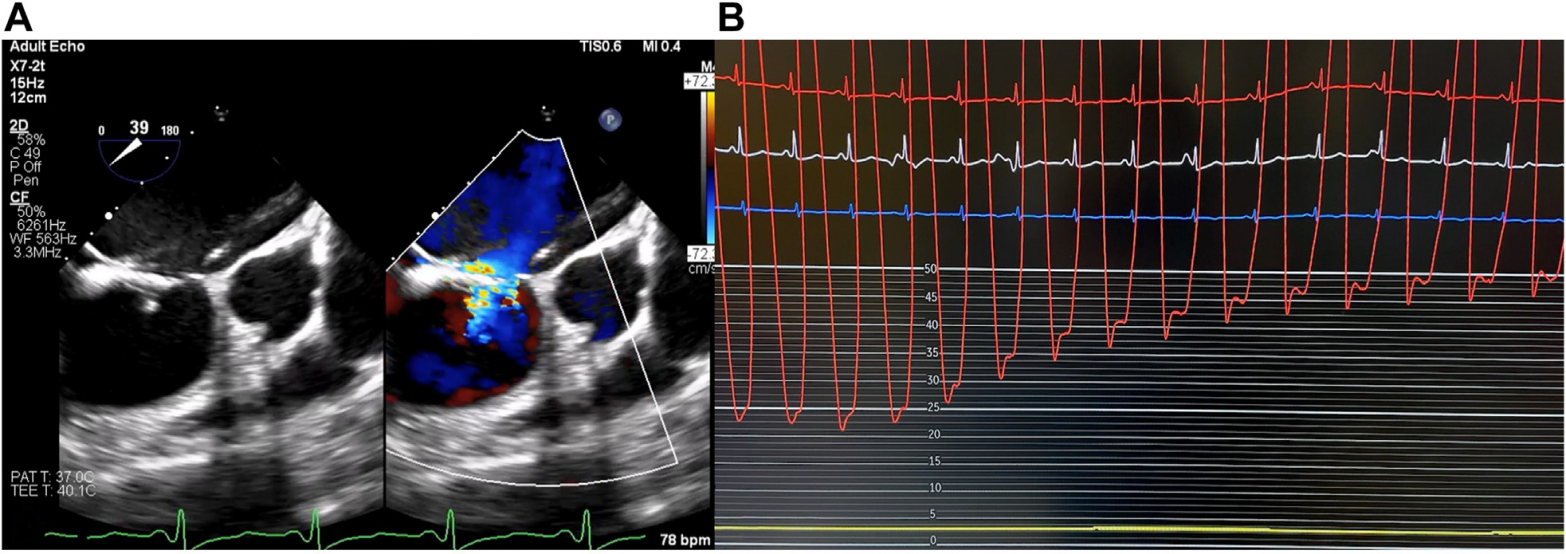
(A) The transesophageal echocardiographic image of partial balloon occlusion of the atrial septal defect, with residual flow around the balloon. (B) The increase in left ventricular end-diastolic pressure with partial balloon occlusion.
